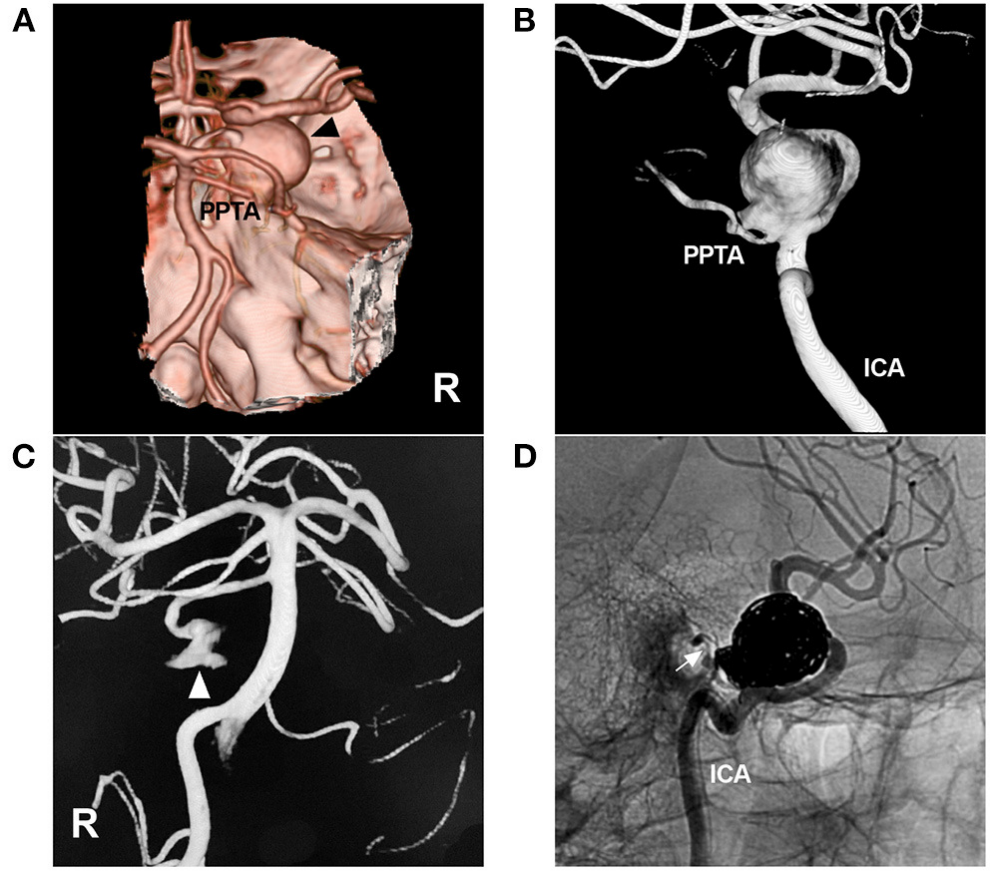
Coiling of an aneurysm of the PPTA-ICA junction. (A): CTA showing a large right parasellar aneurysm (arrowhead); a PPTA can be seen. (B): Three-dimensional DSA of the ICA showing the aneurysm located at the junction of the PPTA and ICA. (C): Three-dimensional DSA of the right VA showing part of the PPTA aneurysm (arrowhead). (D): Unsubtracted DSA showing the aneurysm coiled via the ICA, preserving the PPTA (arrow). CTA, computed tomography angiography; DSA, digital subtraction angiography; ICA, internal carotid artery; PPTA, persistent primitive trigeminal artery; R, right; VA, vertebral artery.
